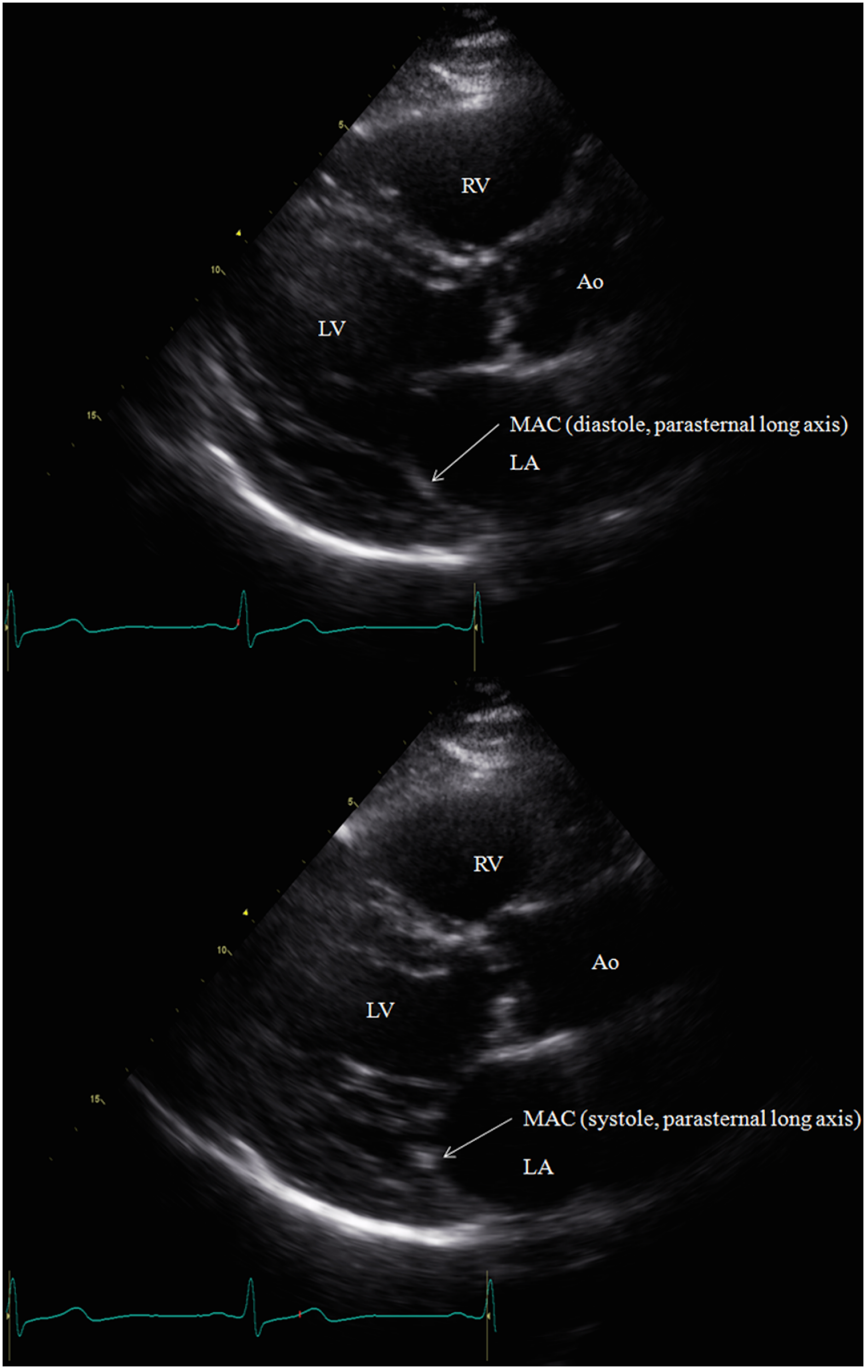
(A-B). MAC was defined as an echodense area visualized throughout systole and diastole, distinguishable from the posterior mitral valve leaflet, located anterior and parallel to the posterior left ventricular wall, seen here in the parasternal long axis view during diastole (A) and systole (B). Abbreviations: Right Ventricle (RV), Left Ventricle (LV), Aorta (Ao), Mitral Annular Calcification (MAC), Left Atrium (LA).
